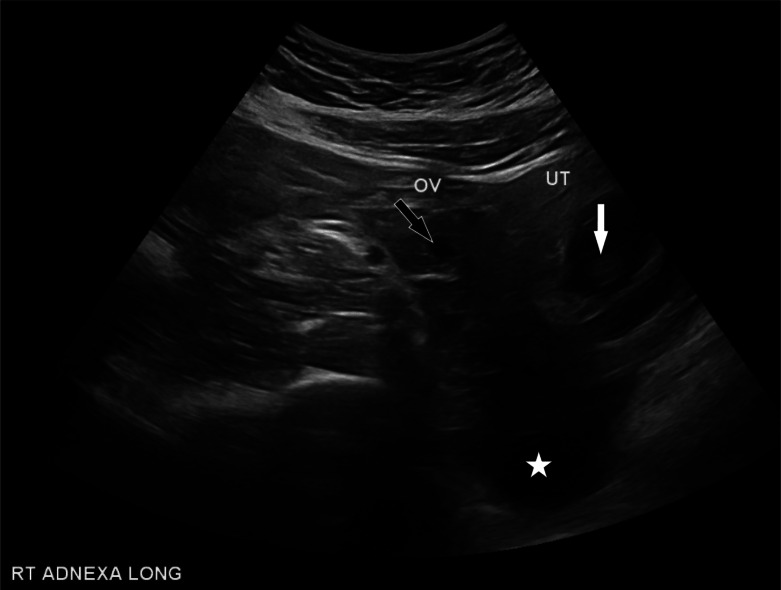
Transvaginal ultrasound showing intrauterine pregnancy (white arrow), right ovary with adjacent cystic structure (black arrow), and fluid in the cul-de-sac (white star).
